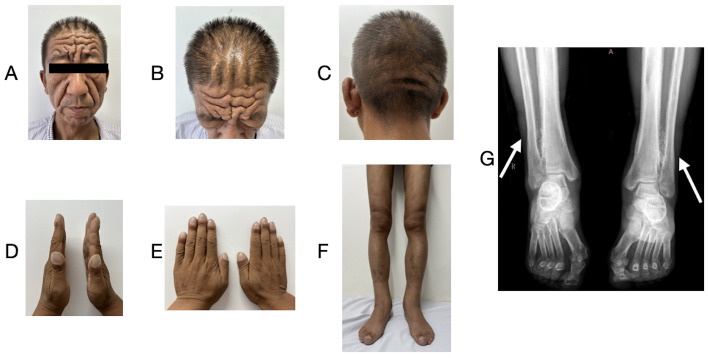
Clinical manifestations of the proband. (A-C) Pachydermia with prominent folds on the front, top and back of the head. (D, E) Digital clubbing. (F) Knee and ankle arthropathy and columnar swelling of the lower limbs. (G) X-ray showing periostosis of the lower limbs.
